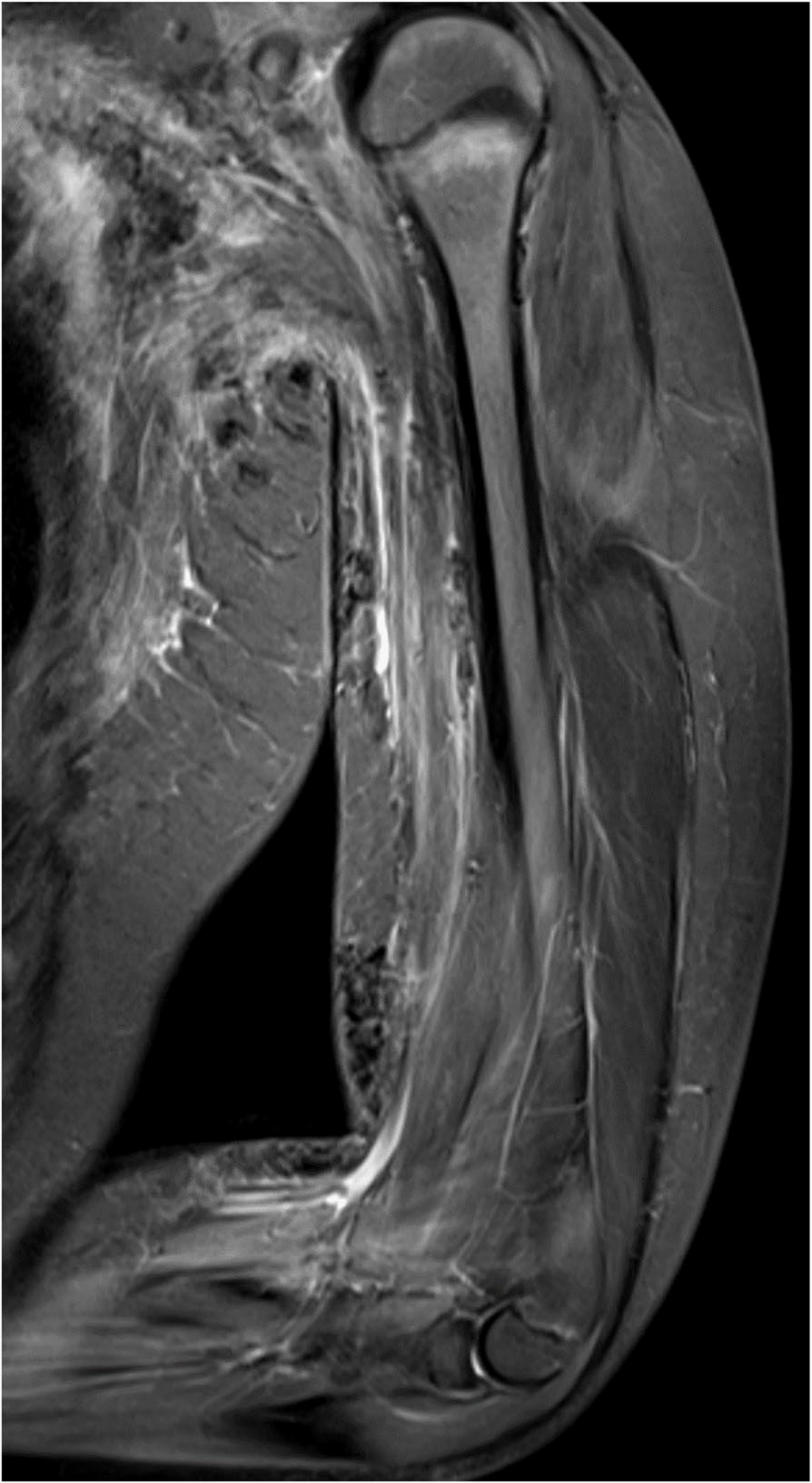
Coronal T2 STIR MRI with multiple areas of hypointensive signal corresponding to extensive lobular subcutaneous calcinosis in a 12-year-old female with juvenile dermatomyositis
